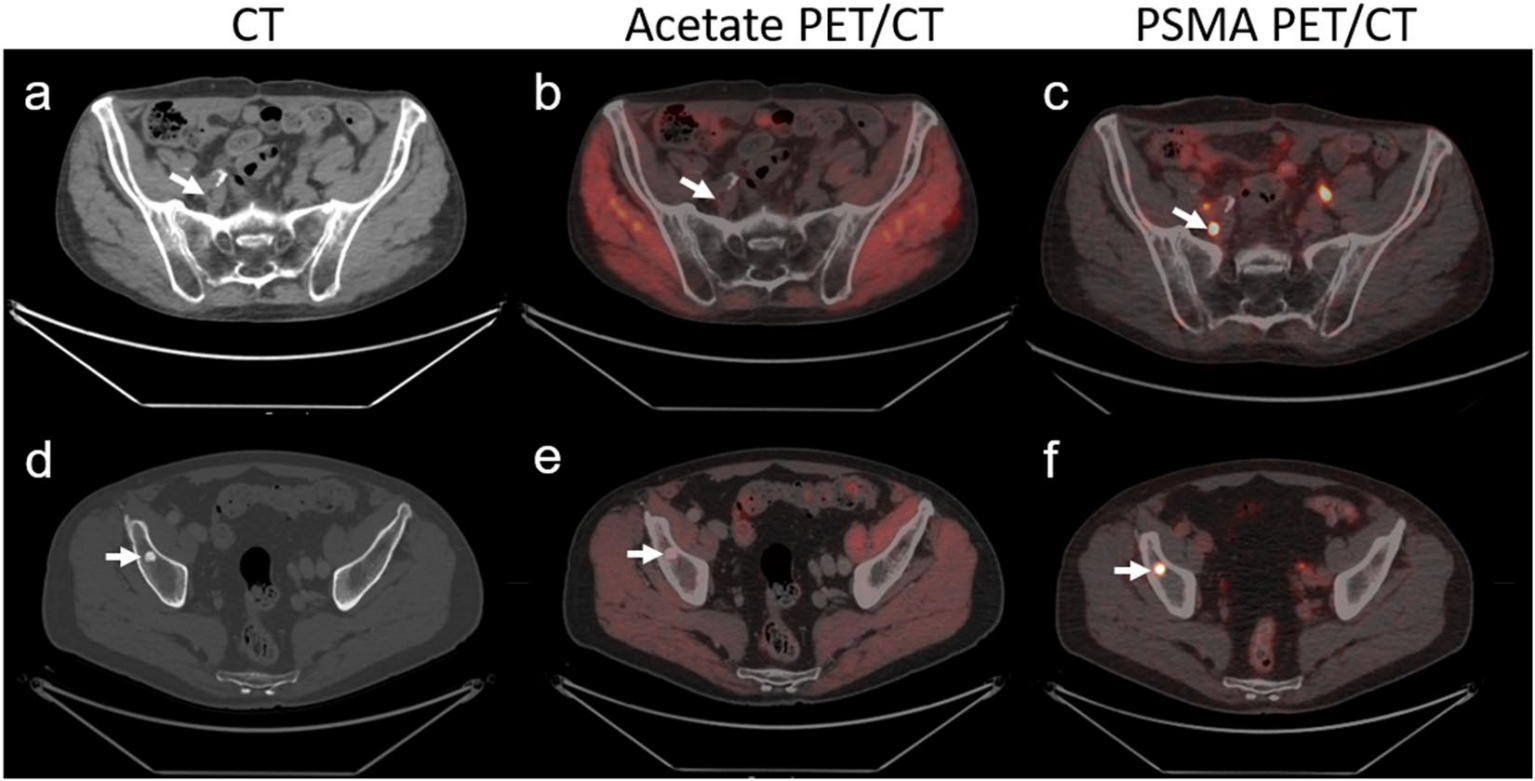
Detection of lymph node (top row) and bone lesions (bottom row) with white arrows on both acetate (b,e) and PMSA PET (c,f) along with CT findings (a,d) in two different subjects. A 76-year-old patient who underwent hormonal and radiation therapy in the past due to PCa (Gleason score 3 + 3). The patient was referred to scans due to PSA elevation 72 ng/mL shows a small lymph node in pelvic region on CT scan a) with no uptake on acetate-PET (b) but with positive lesion on PSMA-PET (c). A 74-year-old patient referred to scan due to a rise in PSA at 28 ng/mL after curative radiation and hormonal therapy to PCa (Gleason score 3 + 4) showing bone lesion on CT (d) with no uptake on acetate-PET (e) but positive on PSMA-PET (f).
